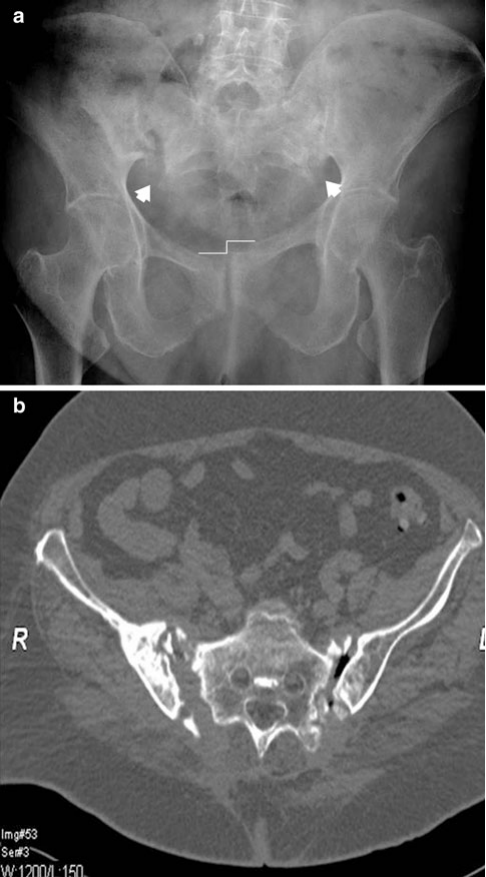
a Bilateral fractures of sacral ala with intrusion of the lumbar spine and sacrum into the small pelvis. There also is instability of the symphysis pubis. b Transverse CT-cuts through the sacrum and dorsal ilium, showing bilateral insufficiency of the sacroiliac joints with widening of the right joint and nitrogen bubble in the left joint
